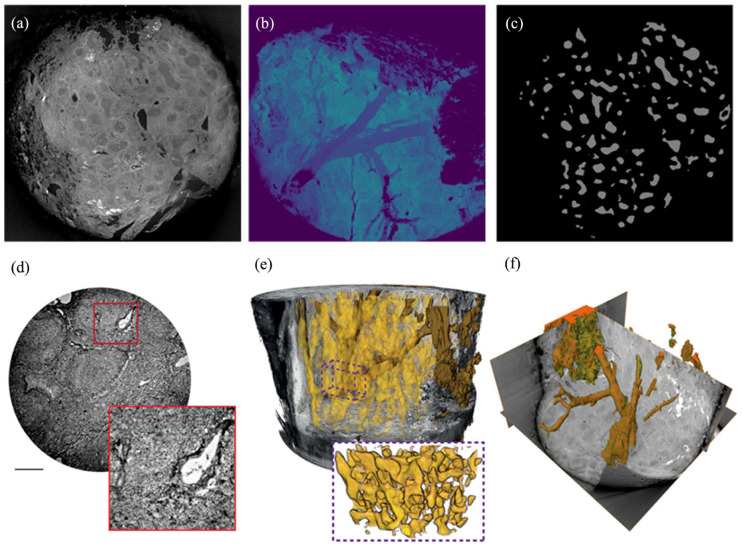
Paraffin-embedded lung tissue was analyzed by multiscale XPCT. (a–c) XPCT-scan could reveal the peribronchial, nodular infiltration of a lymphoid aggregates with GCs (rendered GCs in c) in the BALT. (d) XPCT scan demonstrates the perivascular spreading of lymphoid cells within and surrounding BALT. (e) Segmentation of vascularity is characterized by altered severely abnormal-appearing blood vessels (yellow) which are directly linked to the upstream larger blood vessels (f). XPCT: X-ray phase-contrast computed tomography; GCs: germinal centers; BALT: bronchus-associated lymphoid tissue.
